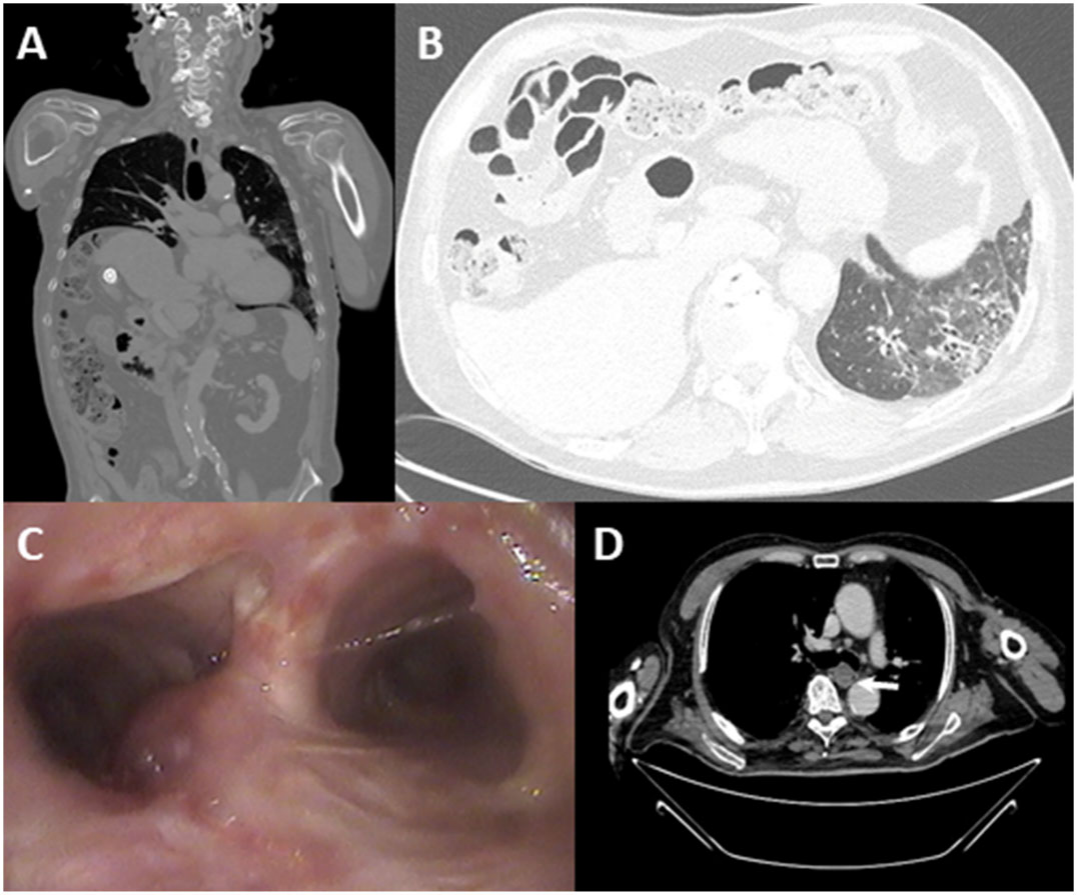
(A) Right hemidiaphragm elevation; (B) left lung fibrosis; (C, D) bronchoscopy and computed tomography images of chondrosarcoma.
